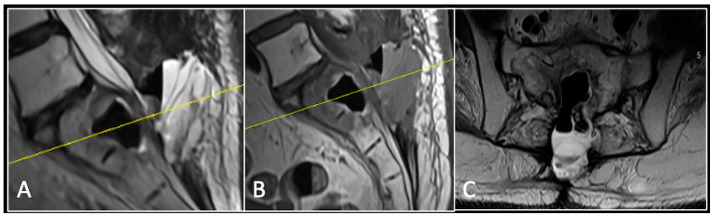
MRI imaging following revision debulking surgery. (A) T2W sagittal; (B) T1W sagittal; (C) T2-weighted axial. Imaging shows an air cavity within the S1 vertebral body where tumour has been resected and fluid within the epidural space that is displacing the thecal sac posteriorly. The yellow line on the sagittal images denotes the level corresponding to the axial section.
